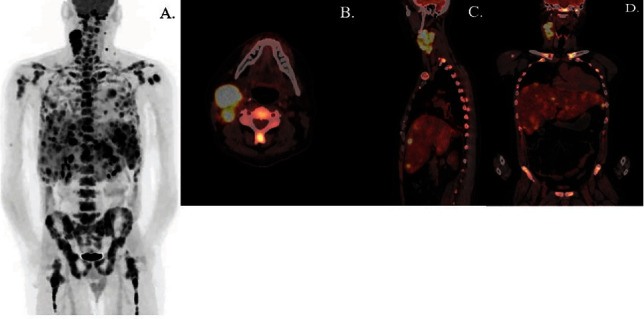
Metastatic involvement demonstrated as PET scan showed (A) cellular activity and fused PET/CT scan showed (B) axial, (C) sagittal, and (D) coronal images of localized high cell activity. Specifically, there was asymmetric hypermetabolic thickening along the right nasopharynx, extensive hypermetabolic right greater than left neck lymphadenopathy consistent with nodal metastases, widespread hypermetabolic osseous disease and marrow infiltration through the axial and appendicular skeleton with innumerable lesions, and extensive hypermetabolic hepatic, splenic, and pulmonary metastatic disease.
